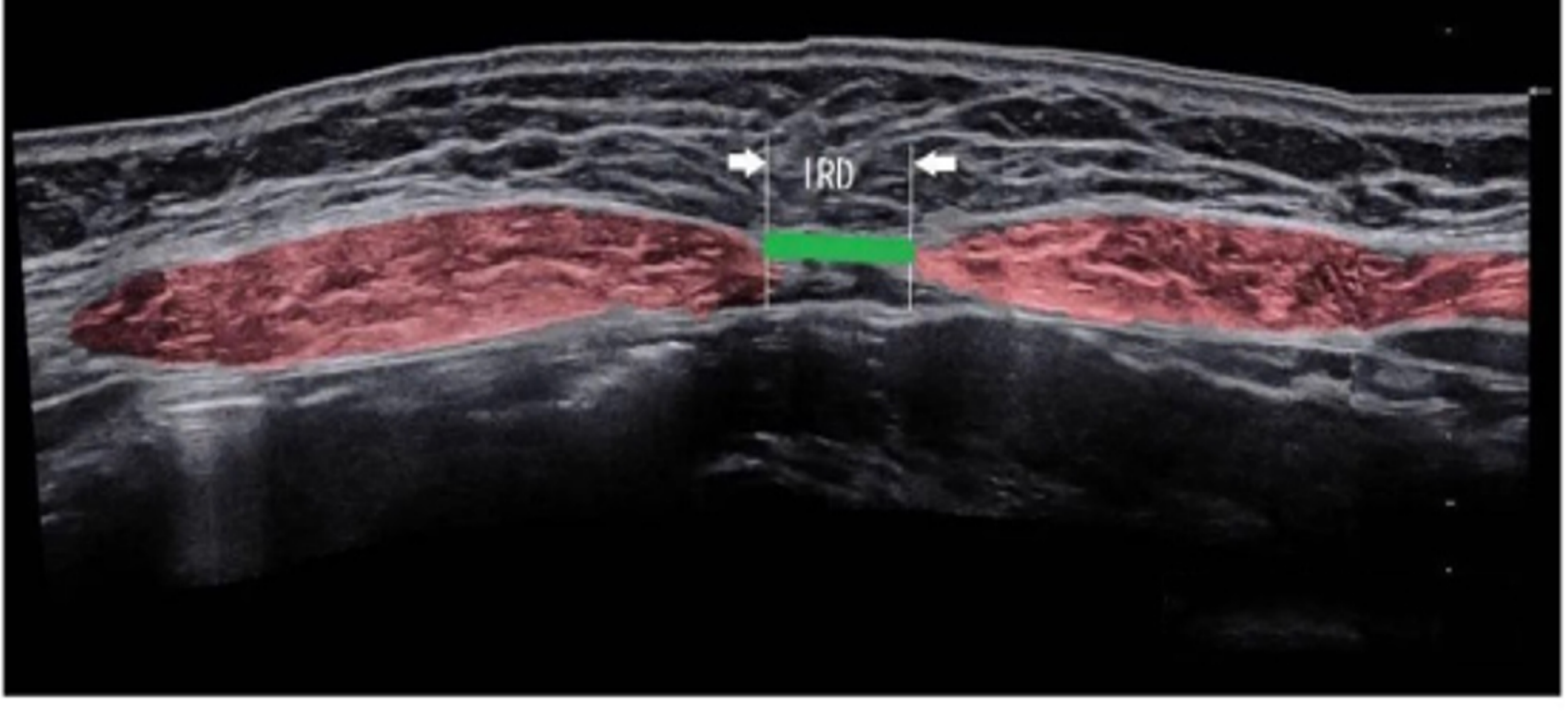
Ultrasound images of diastasis recti abdominis.
